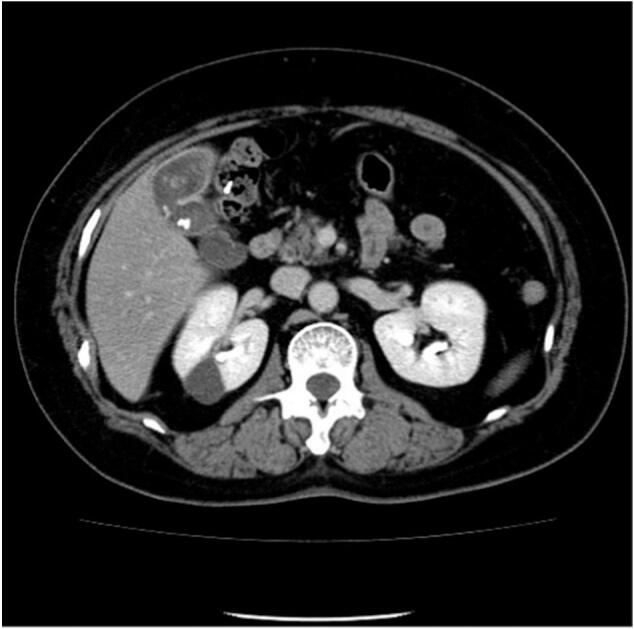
Computed tomography imaging taken 7 years before surgery.
